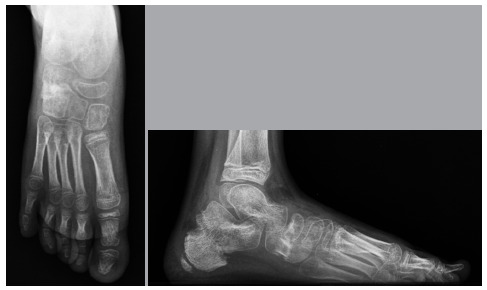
A: Anteroposterior post-op radiograph; B: Lateral radiograph.
